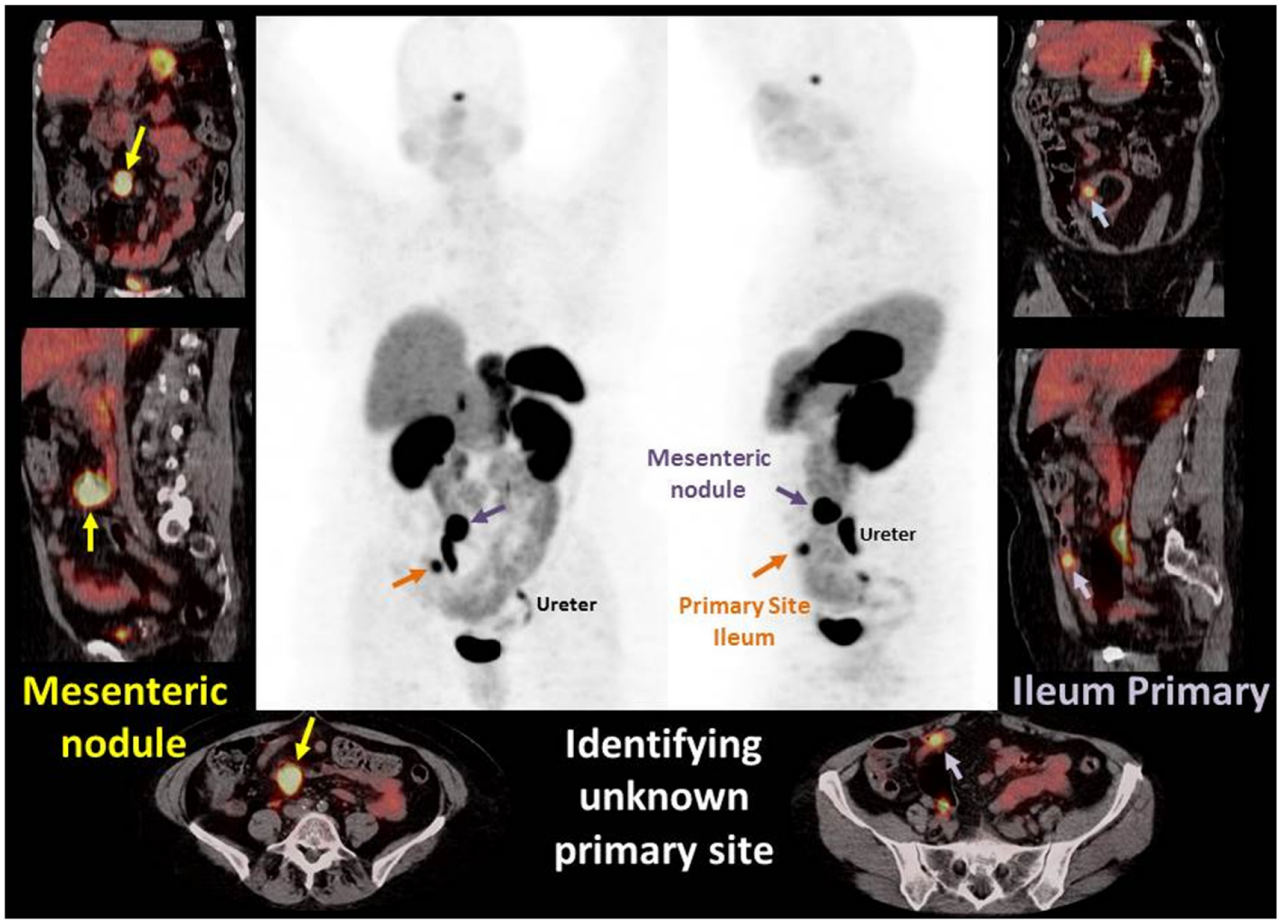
Images demonstrate PET MIP images and fused PET/CT axial, sagittal, and coronal images of a case example of gallium-68 DOTATATE identifying unknown primary site. A 70 year old female patient presented to the emergency department with abdominal pain. Contrast CT of the abdomen and pelvis showed an irregular mesenteric mass and no primary identified. Gallium-68DOTATATE PET/CT demonstrated an avid mesenteric mass and identified an avid ileal primary. Subsequently the patient underwent exploratory laparotomy with small bowel resection and mesenteric node dissection. Pathological examination revealed a well differentiated G2 neuroendocrine tumor. This case highlights capability of gallium 68-dotatate imaging in locating the primary tumor which was otherwise missed in anatomic scans (Contrasted CT scan).
